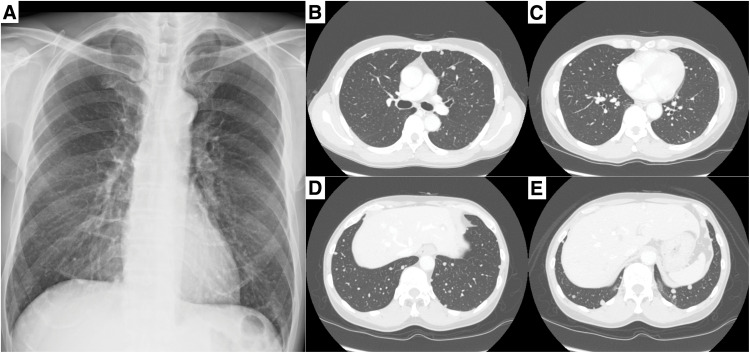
Chest X-ray showing multiple small nodules predominantly in the middle and lower lung fields of both lungs (A). Plain CT revealed multiple well-circumscribed nodules up to 1.0 cm in diameter in the bilateral lungs (B–E).
